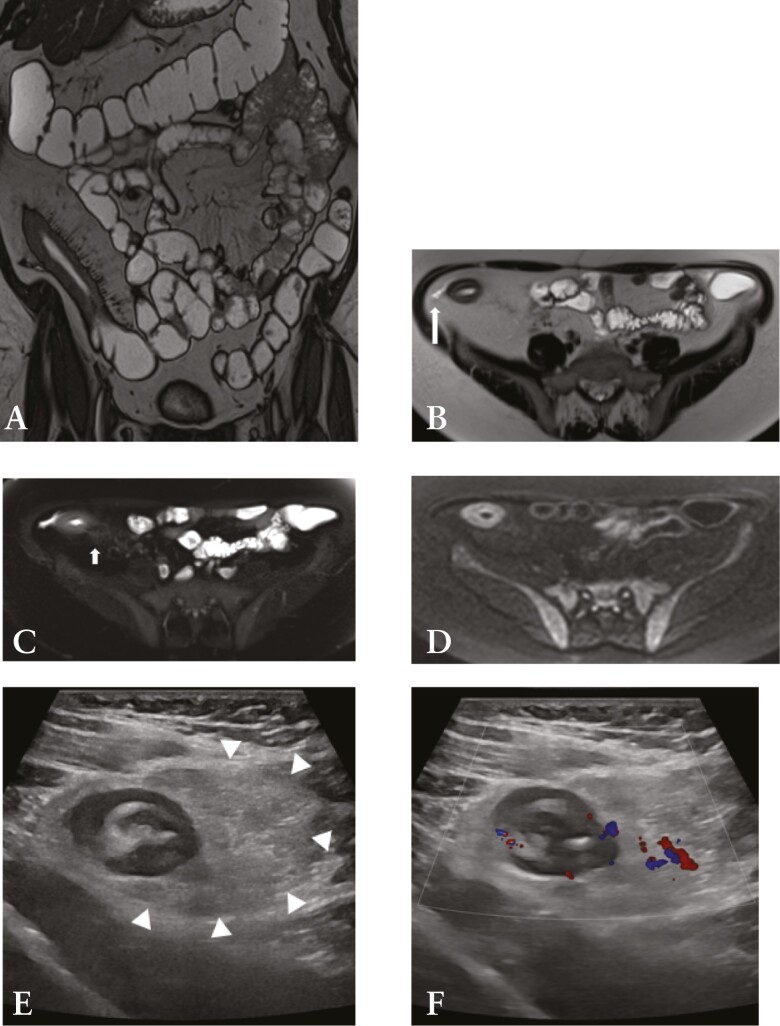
MRE [A–D] and IUS [E–F] images in a 56-year-old female with Crohn’s disease. A: Coronal T2 TRUFI image demonstrating bowel wall thickening at the terminal ileum and engorged vasa recta. B: Axial T2 HASTE image showing mural oedema in the terminal ileum, perienteric fluid [long arrow], and fat wrapping. C: Axial T2 HASTE image with fat saturation facilitates detection of the presence of both mural oedema and mesenteric oedema [short arrow]. D: Axial DWI [b600] image showing restricted diffusion in the inflamed terminal ileum. E: Greyscale IUS image showing bowel wall thickening at the terminal ileum with loss of mural stratification and presence of fat wrapping [arrowheads]. F: Colour Doppler image demonstrating hyperaemia in the thickened terminal ileal wall extending into the hypertrophic mesenteric fat. MRE, magnetic resonance enterography; IUS, intestinal ultrasound; DWI, diffusion-weighted imaging.
